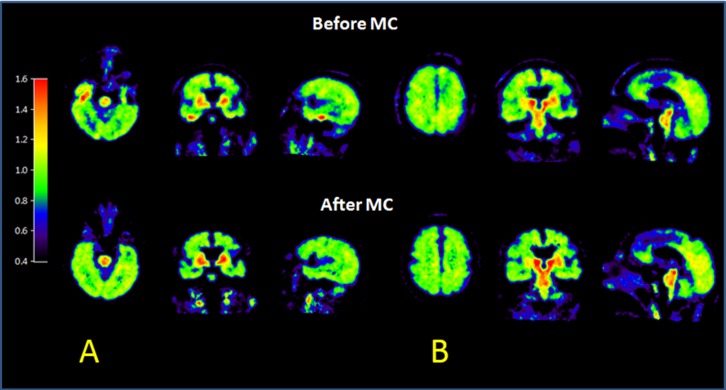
A FDDNP PET/CT scan subject with both head nodding and shaking movement showed reduction in artifacts in the temporal region, reduction in left-right asymmetry and better hemisphere separation after MC. Panels A and B show different brain slices of the same subject before and after MC.
